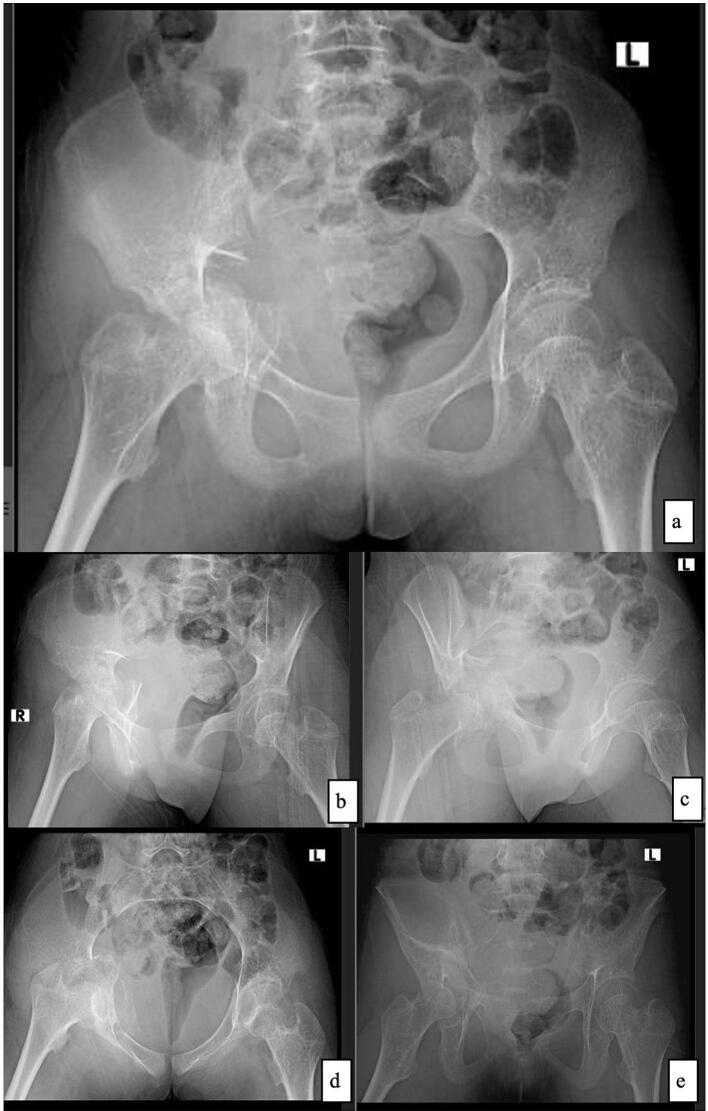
The pre-operative radiographic plain x-ray of the pelvis: (a) AP view, (b) right iliac oblique view, (c) right obturator oblique view, (d) pelvic inlet view, (e) pelvic outlet view showing the discontinuity of acetabular rim with comminuted fracture combined with central dislocation of the femoral head.
